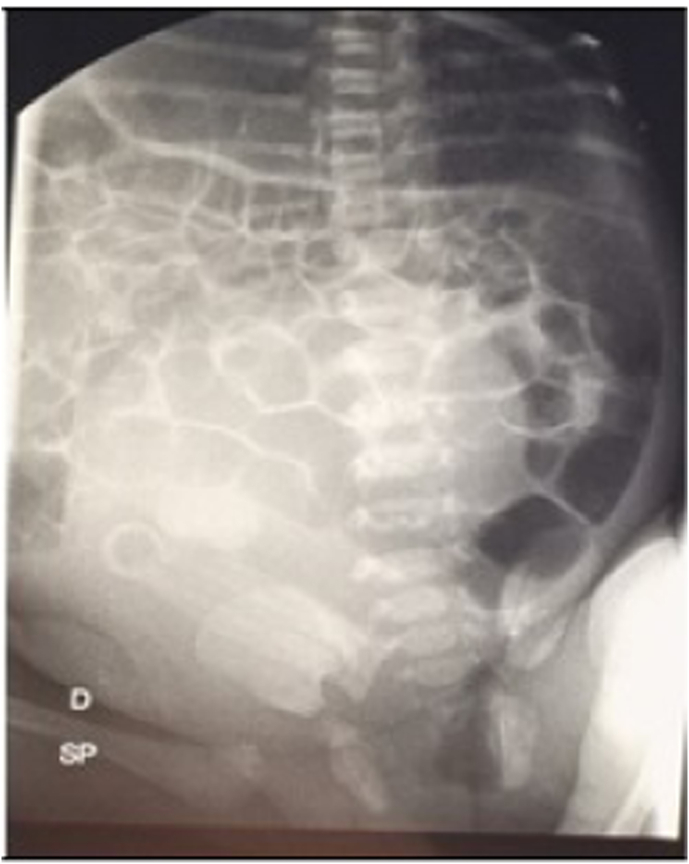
a mass completely filling the lower abdominal cavity visible on abdominal x-ray.
